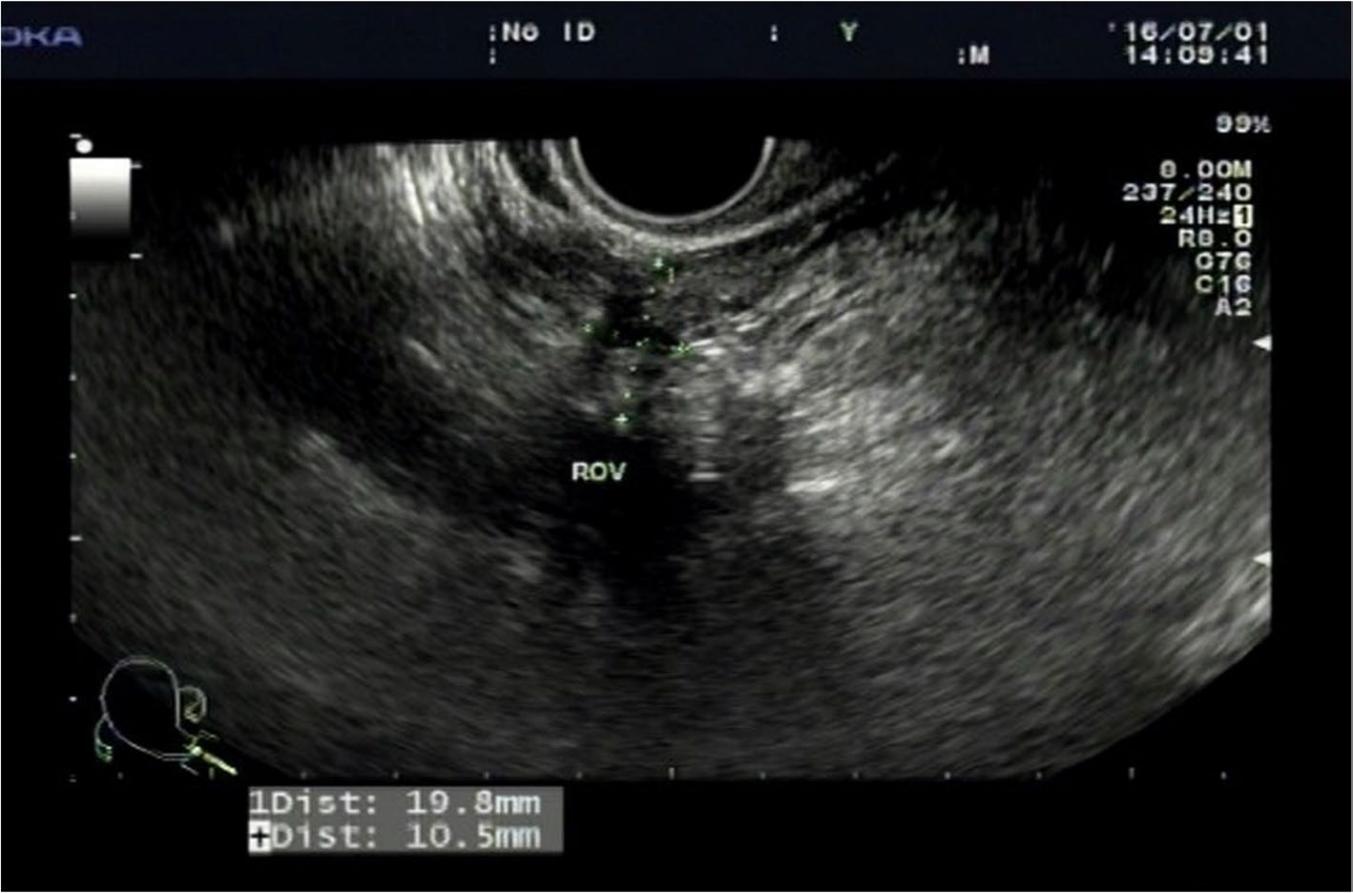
The transvaginal ultrasound image of Case 1: The size of the right ovary was about 19.8 × 10.5 mm, the structure was solid
